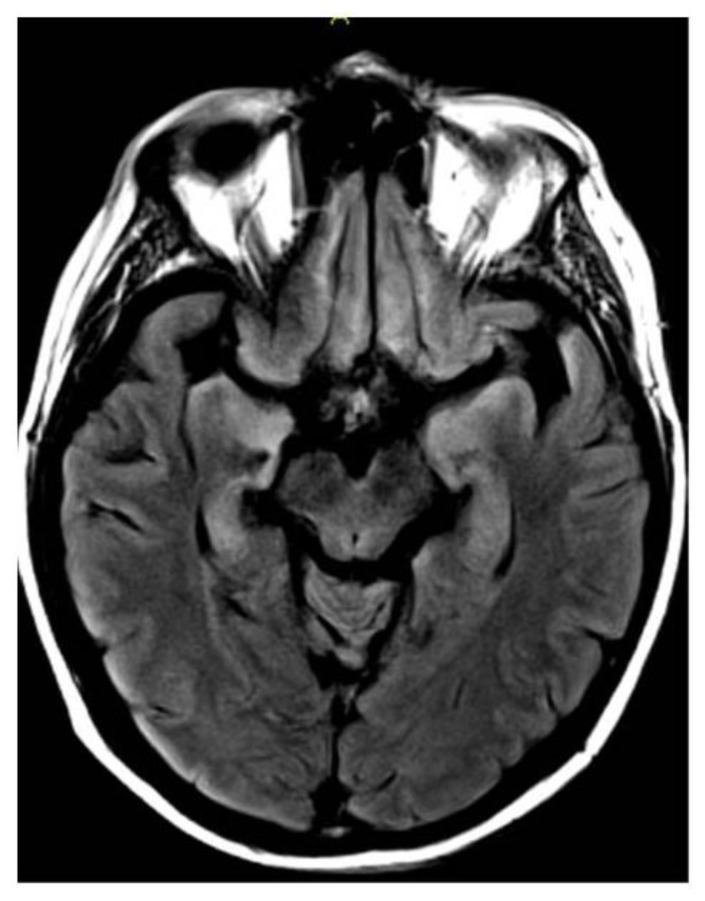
MRI Brain: Increased symmetric T2/FLAIR signal in medial temporal, hippocampi, medial thalamus, and insular regions
